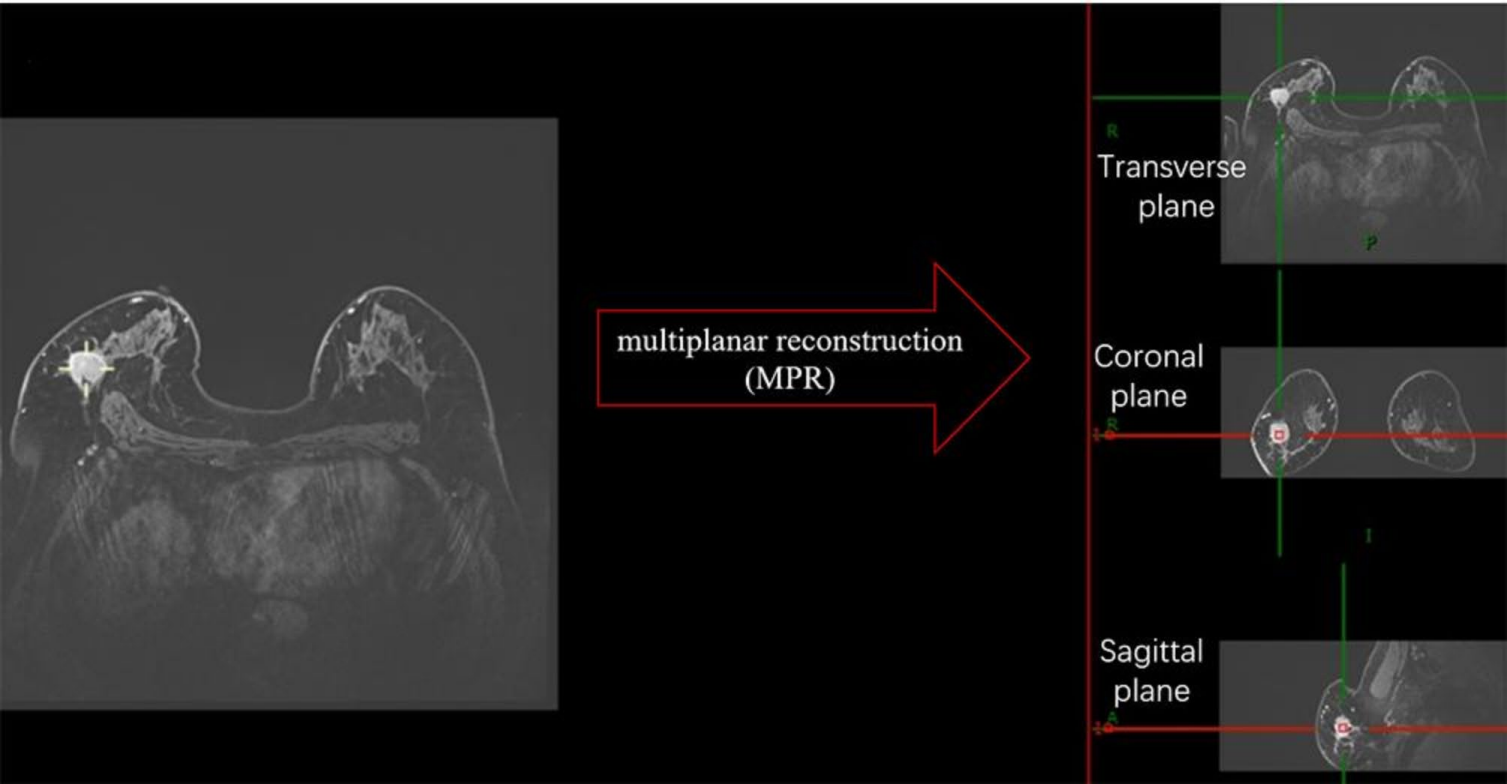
MR images of the same 54-year-old woman with no special type invasive breast cancer: breast tumor size measurements with MRI through multiplanar reconstruction (MPR)
